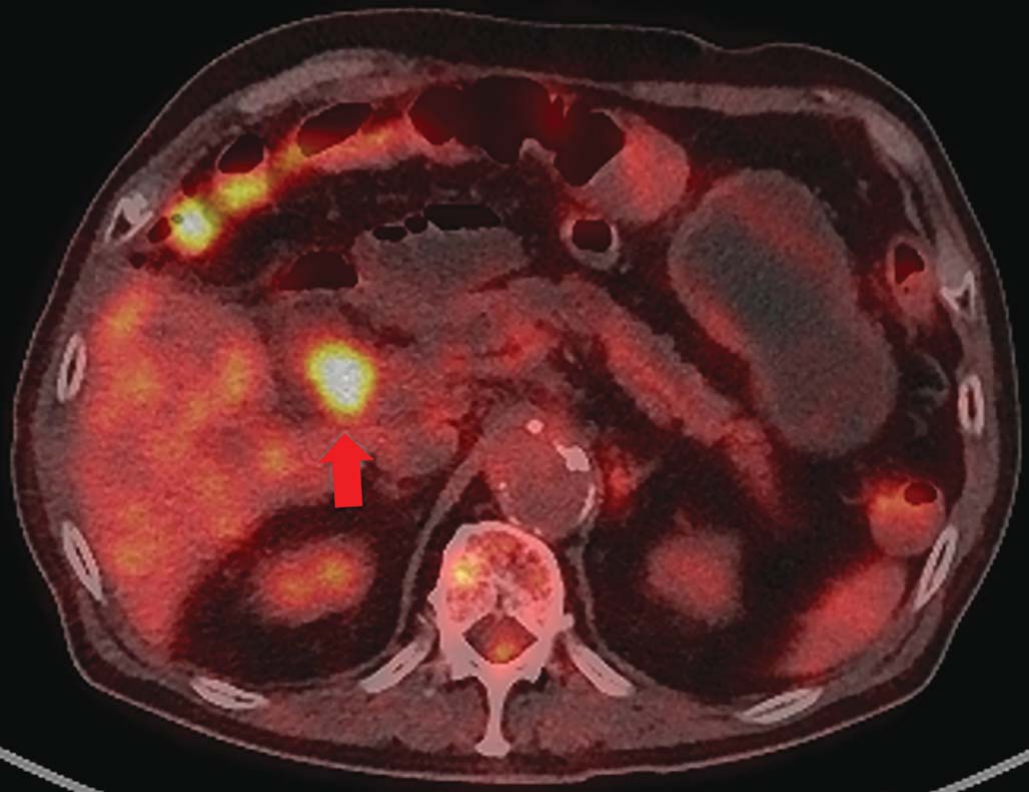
Positron emission tomography–computed tomography shows fluorodeoxyglucose uptake in the intra-abdominal mass at the choledochojejunal anastomotic site (CAS). A red arrow shows an intra-abdominal mass at the CAS with fluorodeoxyglucose uptake.
